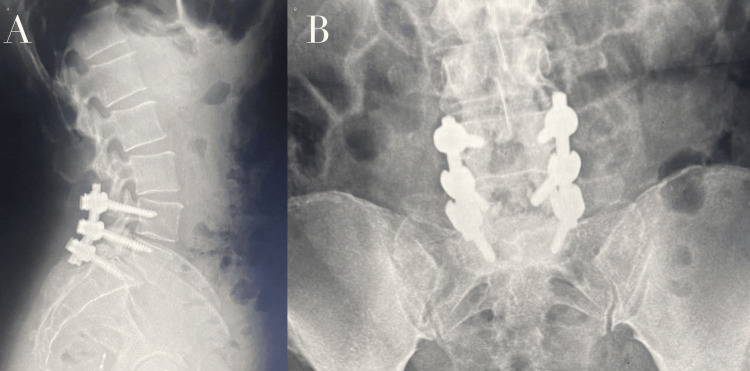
Postoperative imaging after instrumented posterior fusion at L5-S1 (A) Lateral X-ray showing the final construct with pedicle screw fixation and restoration of alignment. (B) Anteroposterior pelvic radiograph confirming appropriate positioning of the fixation hardware at L5-S1.
